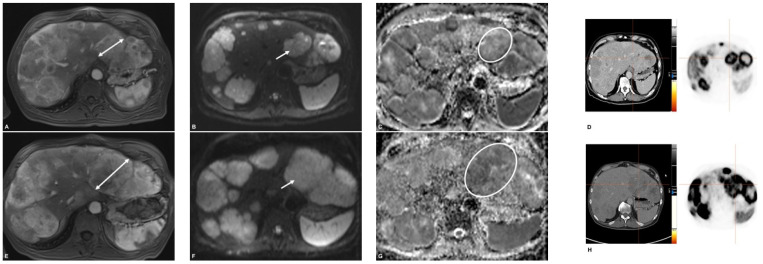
Patient with progressive disease. On arterial phase, lesion in the left lobe showed strong arterial enhancement (A) with diffusion restriction on diffusion-weighted image with b = 800 mm²/s (B) and low signal on ADC map (C). On PET/CT the lesion showed an SSR-overexpression (D). After TARE, there was a significant increase in size of this lesion with remaining arterial enhancement (E), restricted diffusion (F), low ADC signal (G), and increasing SSR overexpression (H). This lesion was rated as progressive disease.
